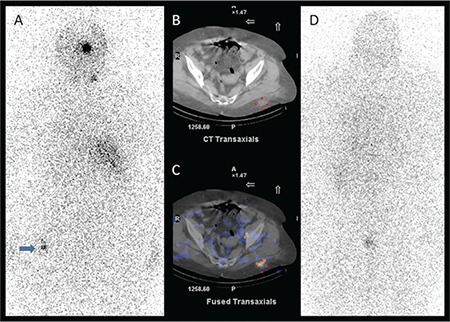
On posterior image of post-therapeutic I-131 whole body scan (A), in addition to residual thyroid tissue, there was a mild focal I-131 accumulation in the pelvic region (arrow). On axial computed tomography (CT) (B) and fused single photon emission computed tomography (SPECT)/CT (C) images, it was clear that the activity was located in the posterior gluteal soft tissue and there were density changes in the fatty tissue due to previous subcutaneous injections. The follow-up diagnostic I-131 scan (D) was normal and the thyroglobulin level was undetectable (<0.2 ng/mL)
